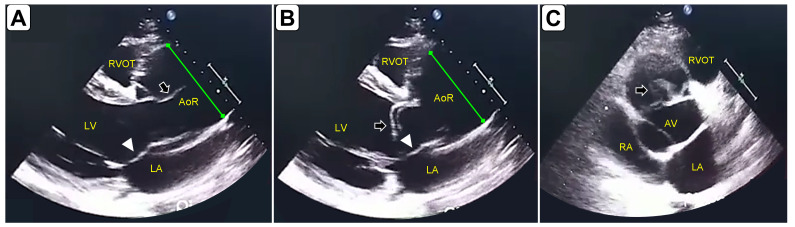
Cardiac point-of-care ultrasound (POCUS) showing signs of type A aortic dissection (A-AD). A. Parasternal long axis (PLAX) view in a systolic frame. B. PLAX view in a diastolic frame. C. Parasternal short-axis view. Arrow indicate the intimal flap, while the continuous green line indicate the aortic root dilation (pseudo-double aortic valve). Arrowhead is pointing to the anterior leaflet of the mitral valve. LV: left ventricle; LA: left atrium; RVOT: right ventricular outflow tract; RA: right atrium;AoR: aortic root; AV: aortic valve.
